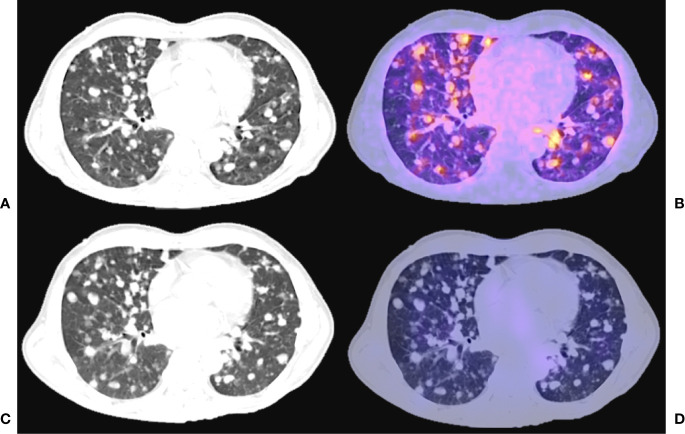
55 years old female, papillary thyroid carcinoma classic variant, treated with thyroidectomy + RAI (22200 MBq cumulative dose). Thyroglobulin 1384 ng/ml. Fused hybrid and simple CT thoracic images. (A) CT acquired for PET fusion (B)
